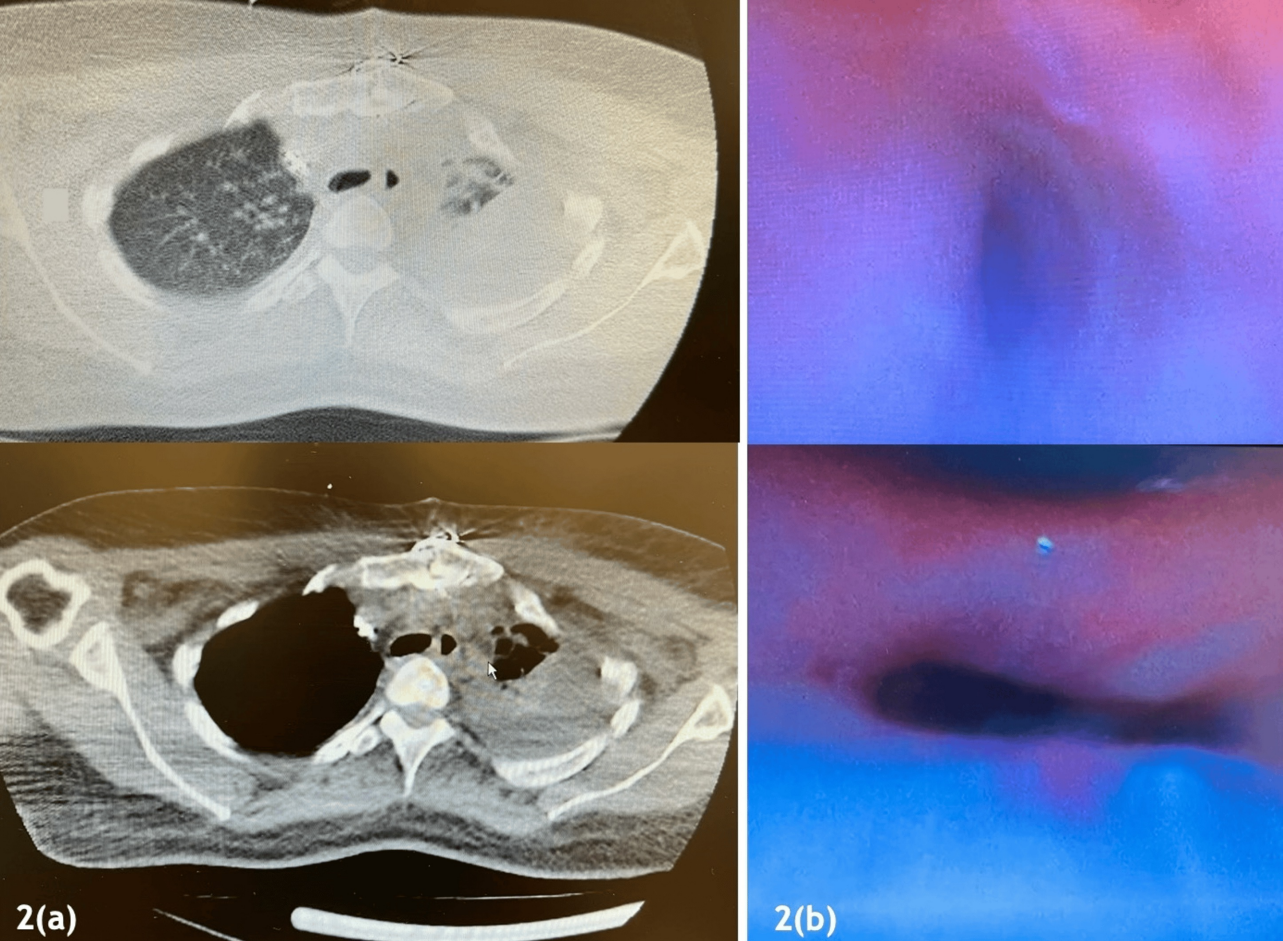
CT (a) and bronchoscopy (b) revealing total collapse of the left lung. (a) Axial chest CT images demonstrate complete collapse of the left lung (arrow), with near-total opacification and marked volume loss, and compensatory hyperinflation of the right lung. (b) Bronchoscopic images reveal complete obstruction of the left main bronchus.
